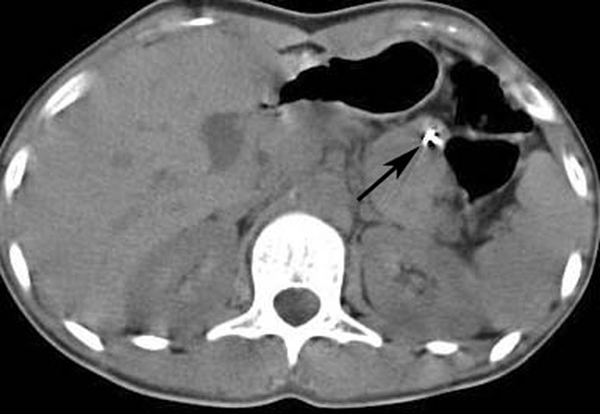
Axial non-enhanced CT scan showed hyperdensity due to metallic sewing needles in the jejunal lumen.
